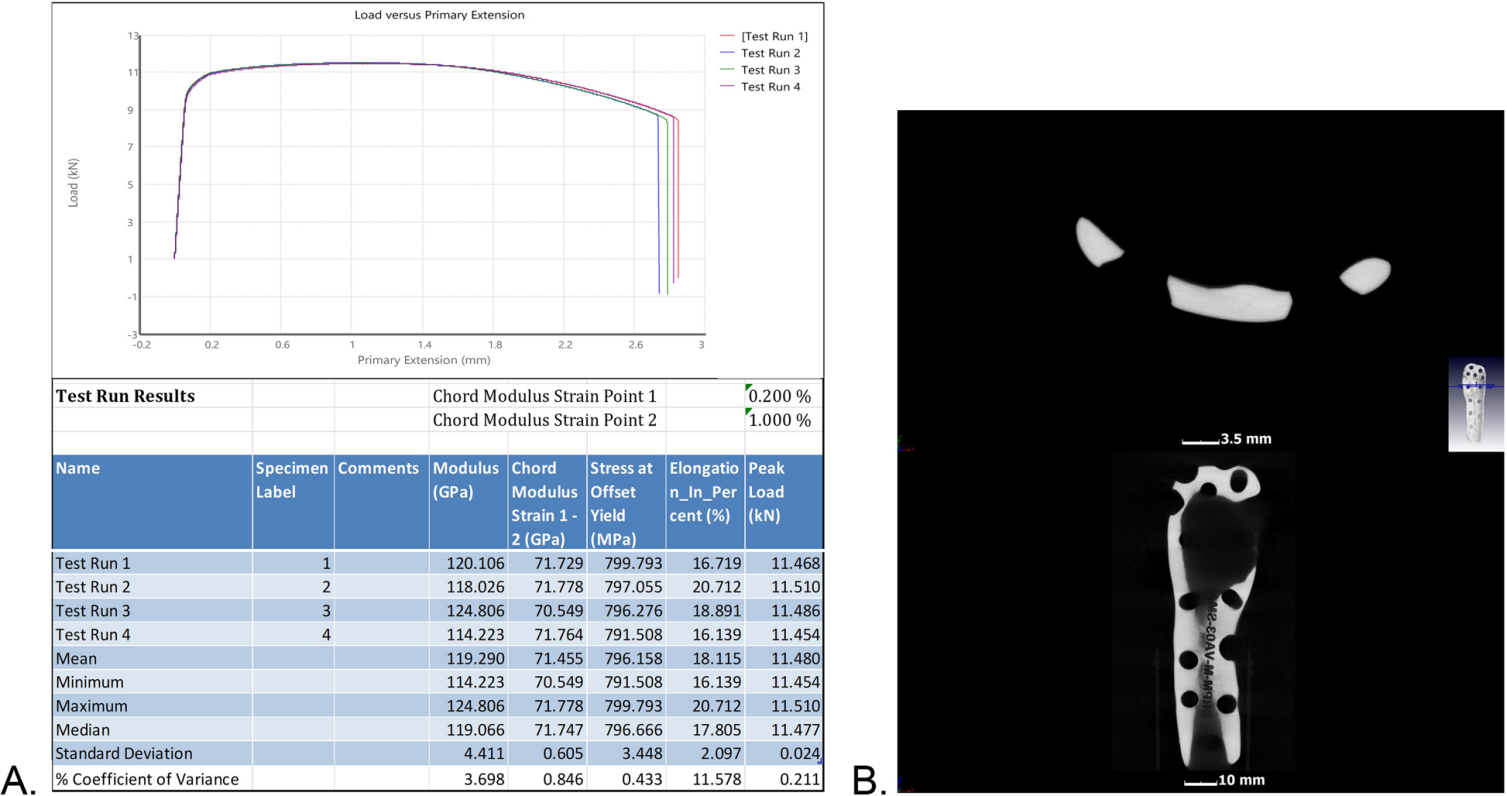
A) graph and table presentation of mechanical properties conducted on the destructive test samples, B) CT scan of implant showing no microcracks after post processing
